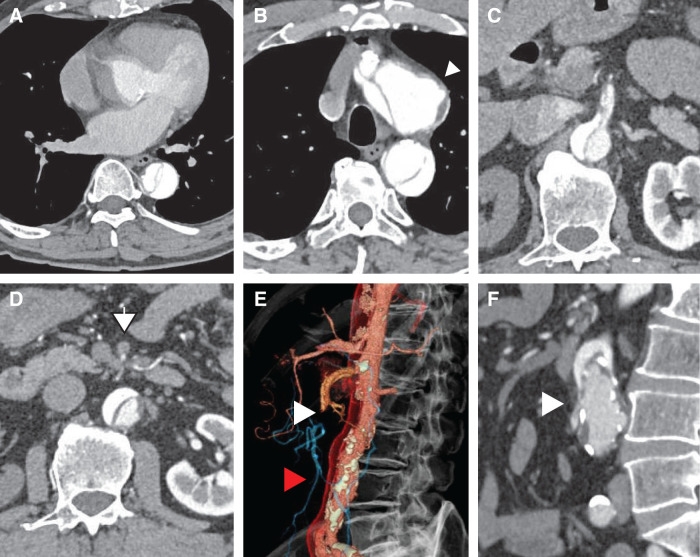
CT findings in preoperative aortic dissection. (A) Thrombosis is present in the false lumen of the ascending aorta. (B) A saccular aneurysm is visible in the distal aortic arch. (C) Dissection involves the SMA origin, demonstrating a characteristic double-barrel appearance with blood flow present in both true and false lumens. (D, E) Complete occlusion of the main SMA trunk (white arrowhead); distal SMA is opacified via collaterals from the IMA (red arrowhead). (F) Calcified stenosis at the IMA ostium with reduced contrast enhancement. IMA, inferior mesenteric artery; SMA, superior mesenteric artery
